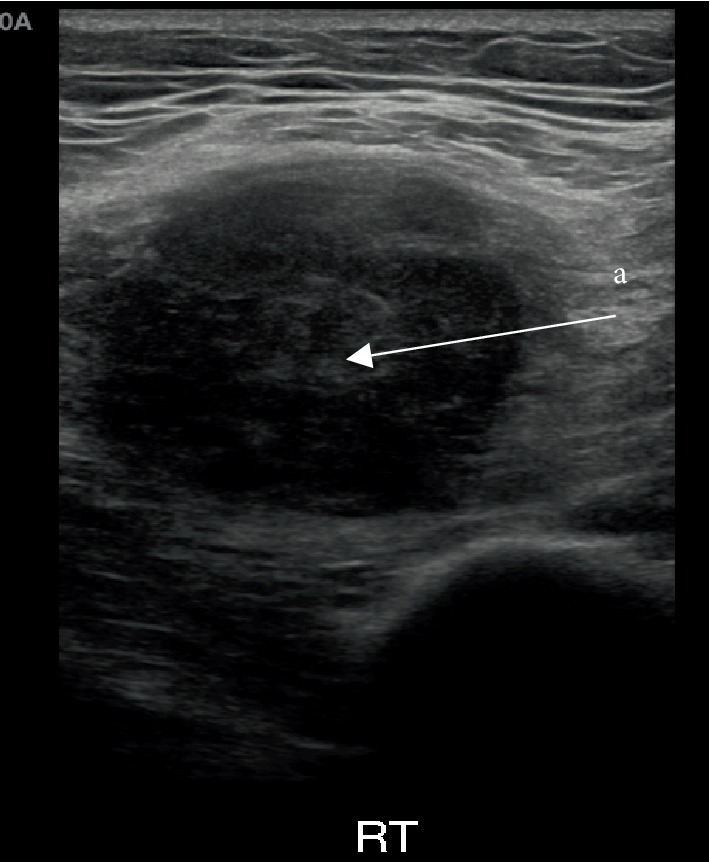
Ultrasound image of a mass in the right vastus lateralis muscle. a: Hypoechoic lesion in right vastus lateralis muscle.
